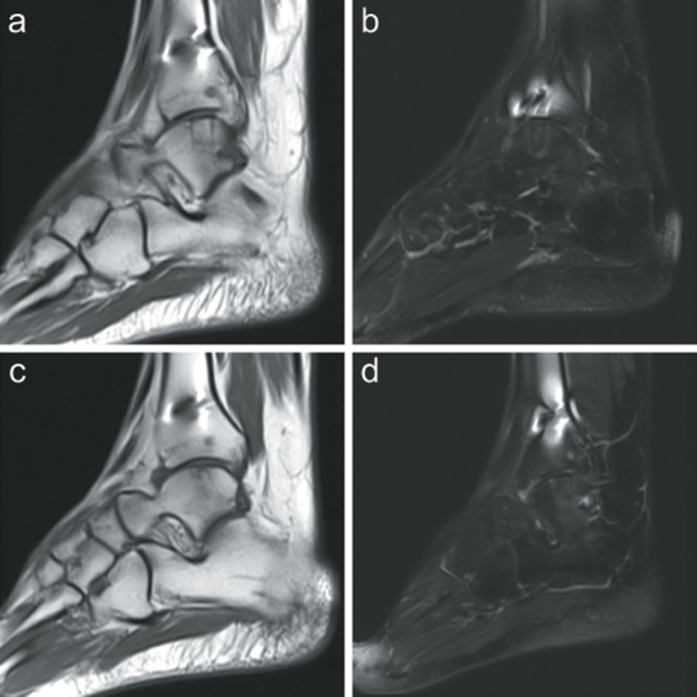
Pre and postoperative radiographs. Preoperative (a) anteroposterior and (b) lateral radiographs. Postoperative 1^st^ year (c) anteroposterior and (d) lateral radiographs.
